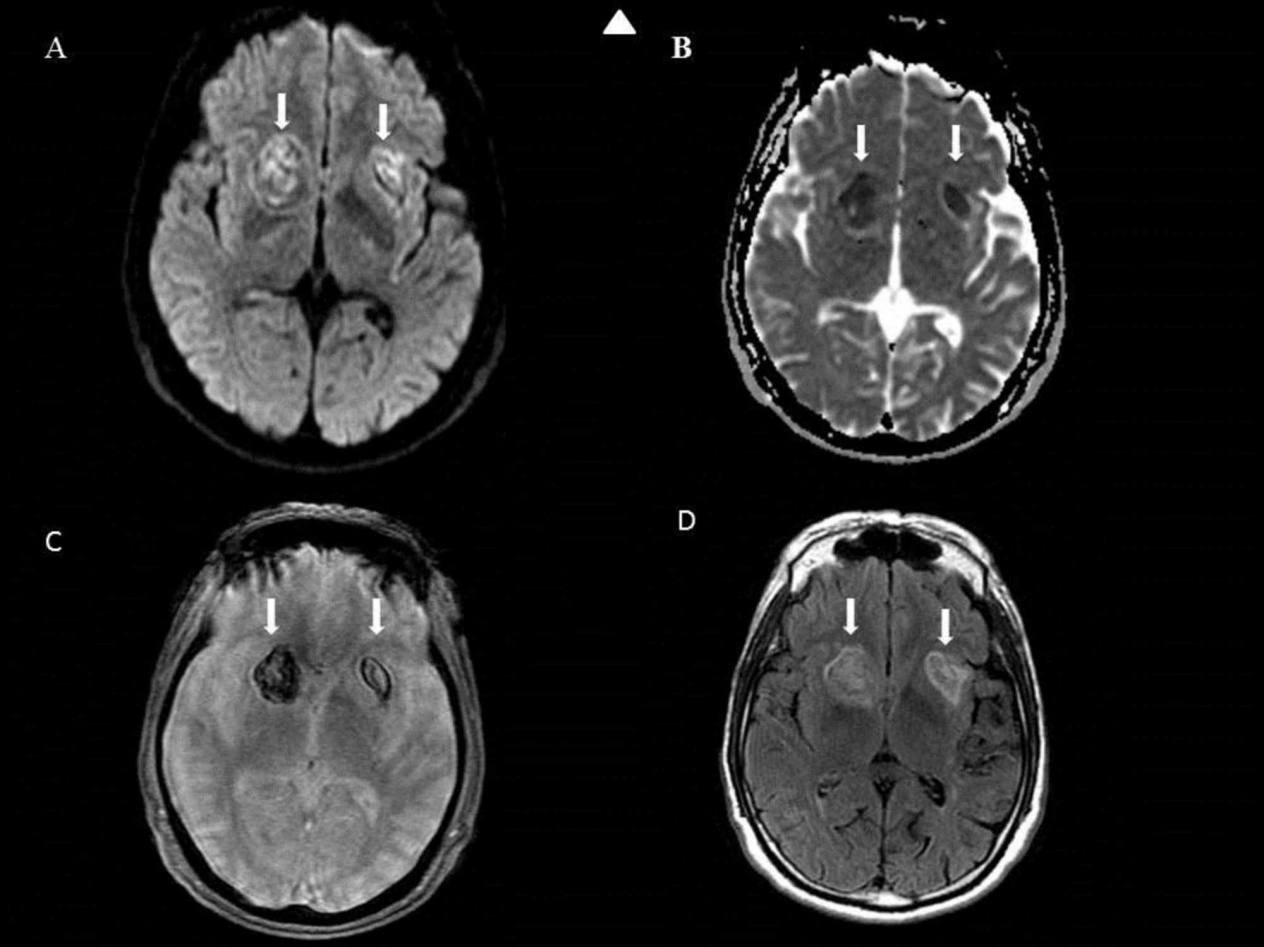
MRI Brain Brain MRI demonstrates 1) no definite evidence of acute ischemic infarct; 2) subacute hematoma in the bilateral basal ganglia greater on the right with extension into the right frontal bone as on the previous CT; 3) small T1 and flair hyperintensity with possible slight enhancement at the posterior aspect of the left Sylvian fissure, possibly a small vascular lesion; 4) evidence of mild to moderate chronic ischemic foci in the white matter. Arrowhead indicates top. Arrows indicate areas of interest on this MRI brain study with and without contrast. Correlates with clinical assessment of hypertensive subacute ICH in bilateral basal ganglia. A: MRI WWO contrast DWI B: MRI WWO contrast ADC C: MRI WWO contrast GRE D: MRI WWO contrast T2 flair ICH - intracerebral hemorrhage; WWO - with and without contrast; DWE - diffusion-weighted images; ADC - apparent diffusion coefficient; GRE - gradient recalled echo
